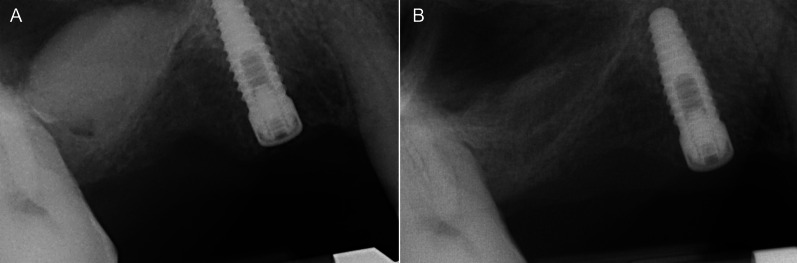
Periapical radiographs taken immediately after transcrestal sinus floor elevation without simultaneous implant insertion (A) and after 6 months of healing (B): an evident shrinkage of the regenerated volume is present
